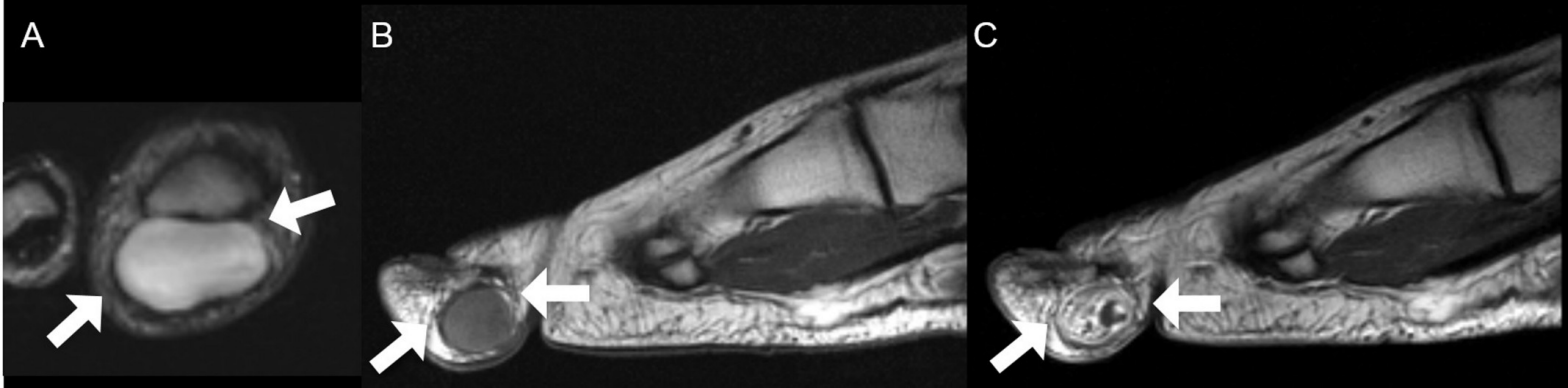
A 15-year-old woman with digital neurofibroma on the plantar side of the 1^st^ distal phalanx of the foot. (A) On axial T2-weighted image, the mass shows mild lobulation and bright SI similar to fluid (arrows). (B) On sagittal T1-weighted image, the mass shows mild hyperintense SI compared to the skeletal muscle (arrows). (C) On sagittal contrast-enhanced T1-weighted image, the mass shows heterogeneously intense enhancement (arrows). This image characteristic correlates well with other reported cases of neurofibroma as iso or slightly hyperintense tissue on T1-weighted images, mildly or markedly hyperintense to skeletal muscle signals on T2-weighted image, and heterogeneous enhancement [18, 19]. *SI*, signal intensity.
